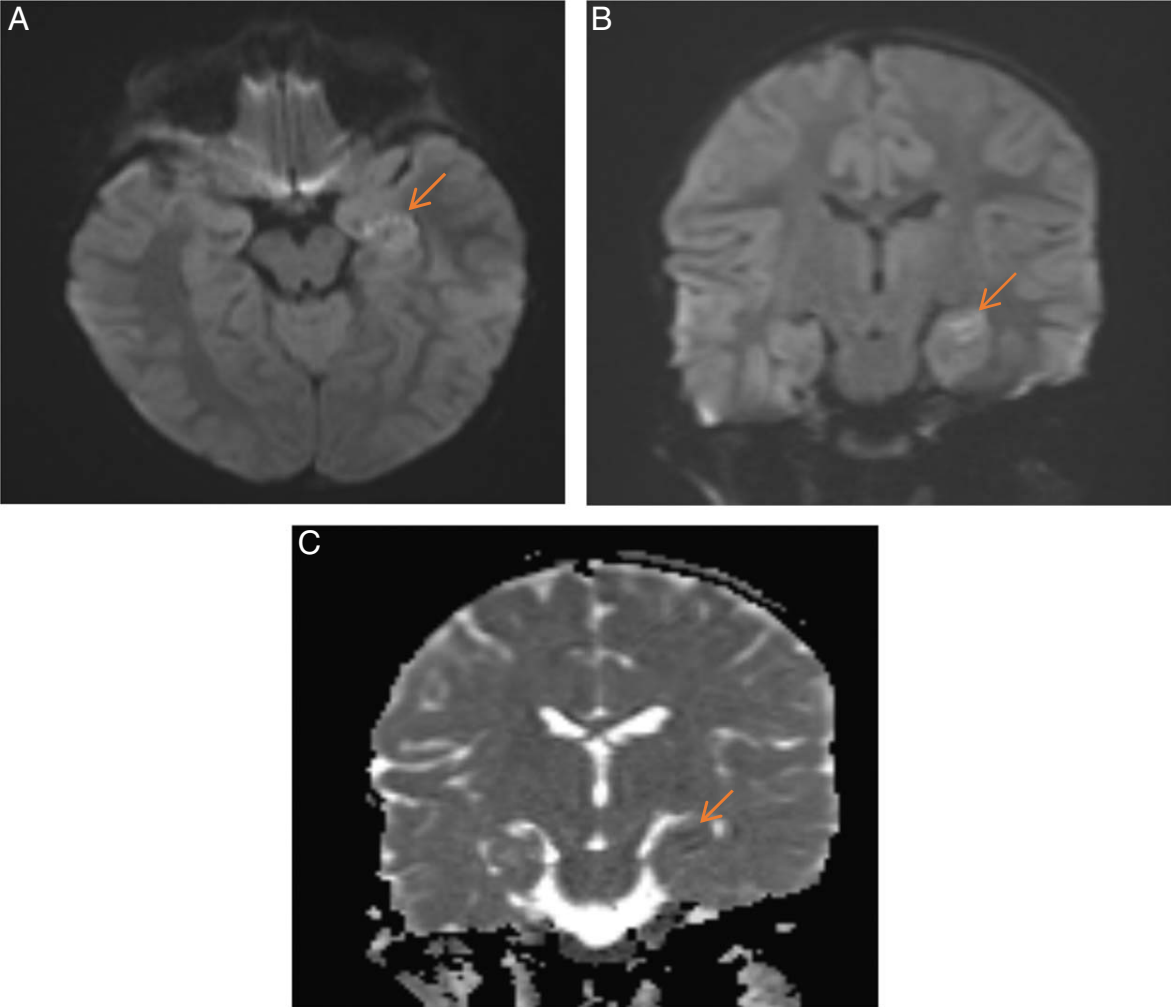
(A) Axial and (B) coronal view showing subtle abnormal signal intensity (abnormal hyperintensity) on the diffusion imaging in the medial left temporal lobe in the region of hippocampal formation. (C) Coronal view apparent diffusion coefficient (ADC) MRI, there is corresponding decrease signal on the ADC maps in this area. Mostly limbic encephalitis, there is no evidence of acute infraction or haemorrhage in the remainder of the imaging of the brain. The remainder of the MRI of the brain is within normal limits.
